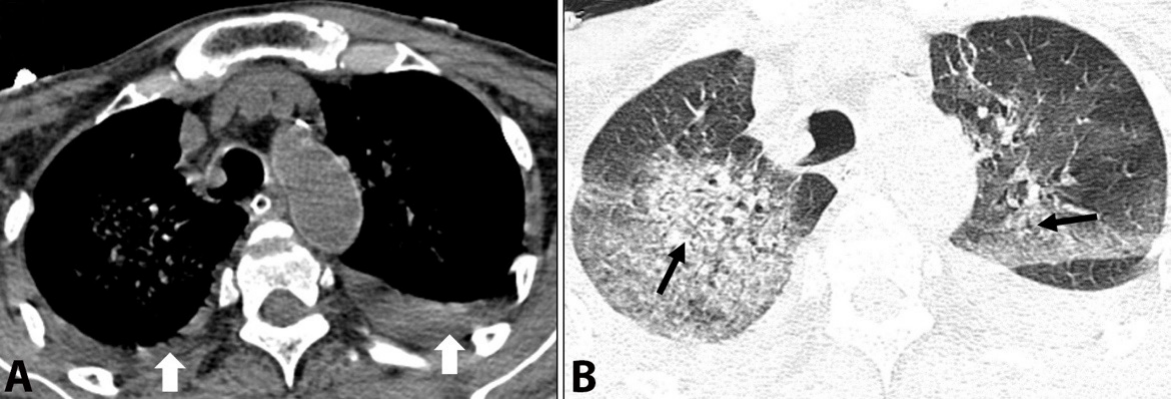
A panel of axial non-contrast thoracic CT scan images showing: A – bilateral small pleural effusions (arrows), in the mediastinal window, and B – bilateral parenchymal infiltrates in lung window.
